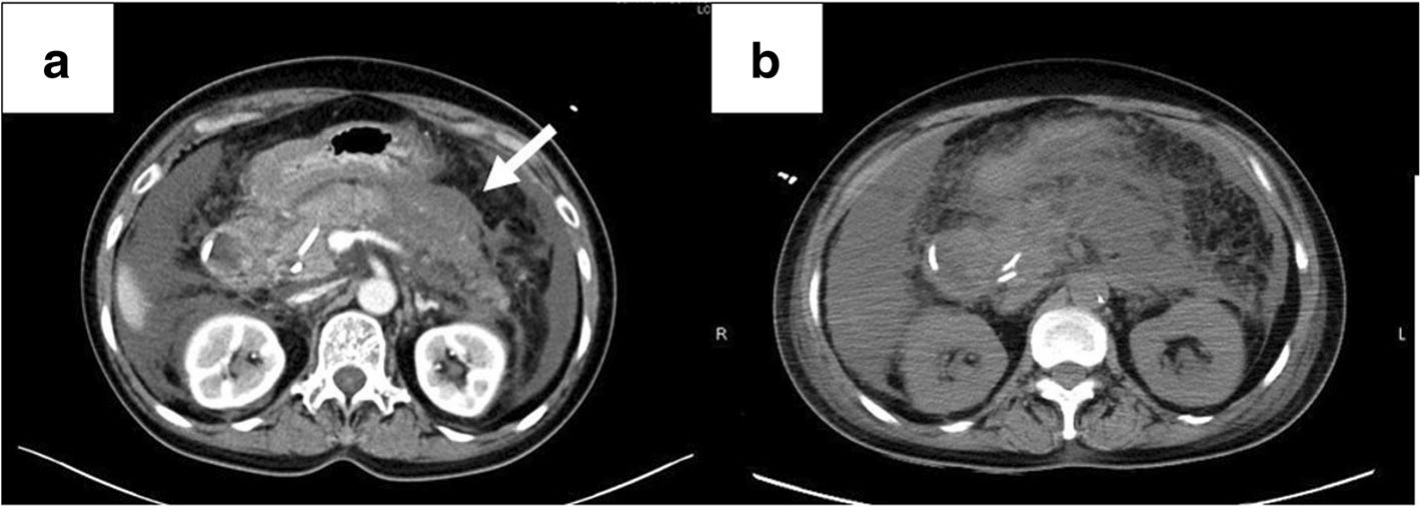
a Abdominal contrast-enhanced CT showed contrast failure (white arrow) and extension of surrounding inflammation on the 2nd hospital day. b Abdominal CT revealed progression of pancreatic enlargement and paralytic ileus on the 5th hospital day
